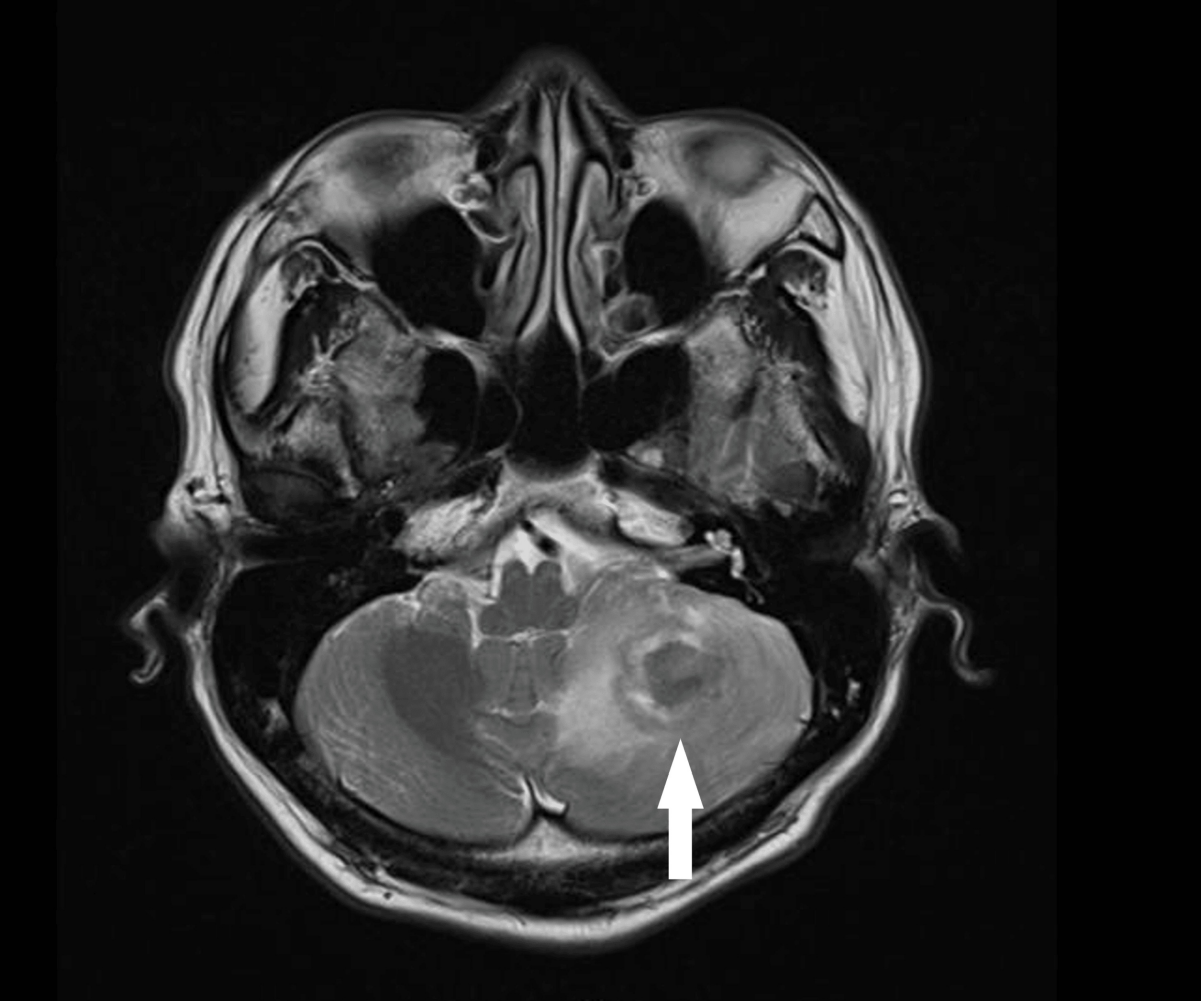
Axial-view T1-weighted image showing a left cerebellar lesion
